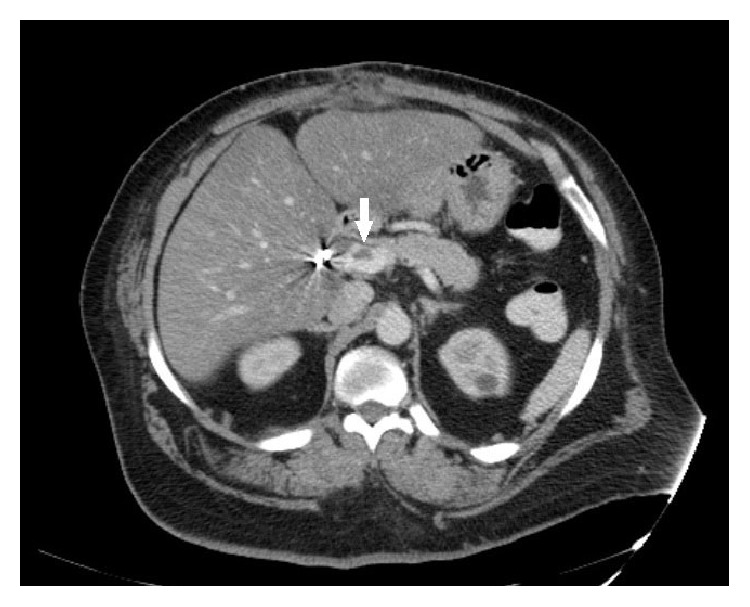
Computed tomography of abdomen and pelvis axial view showing portal vein thrombosis.
